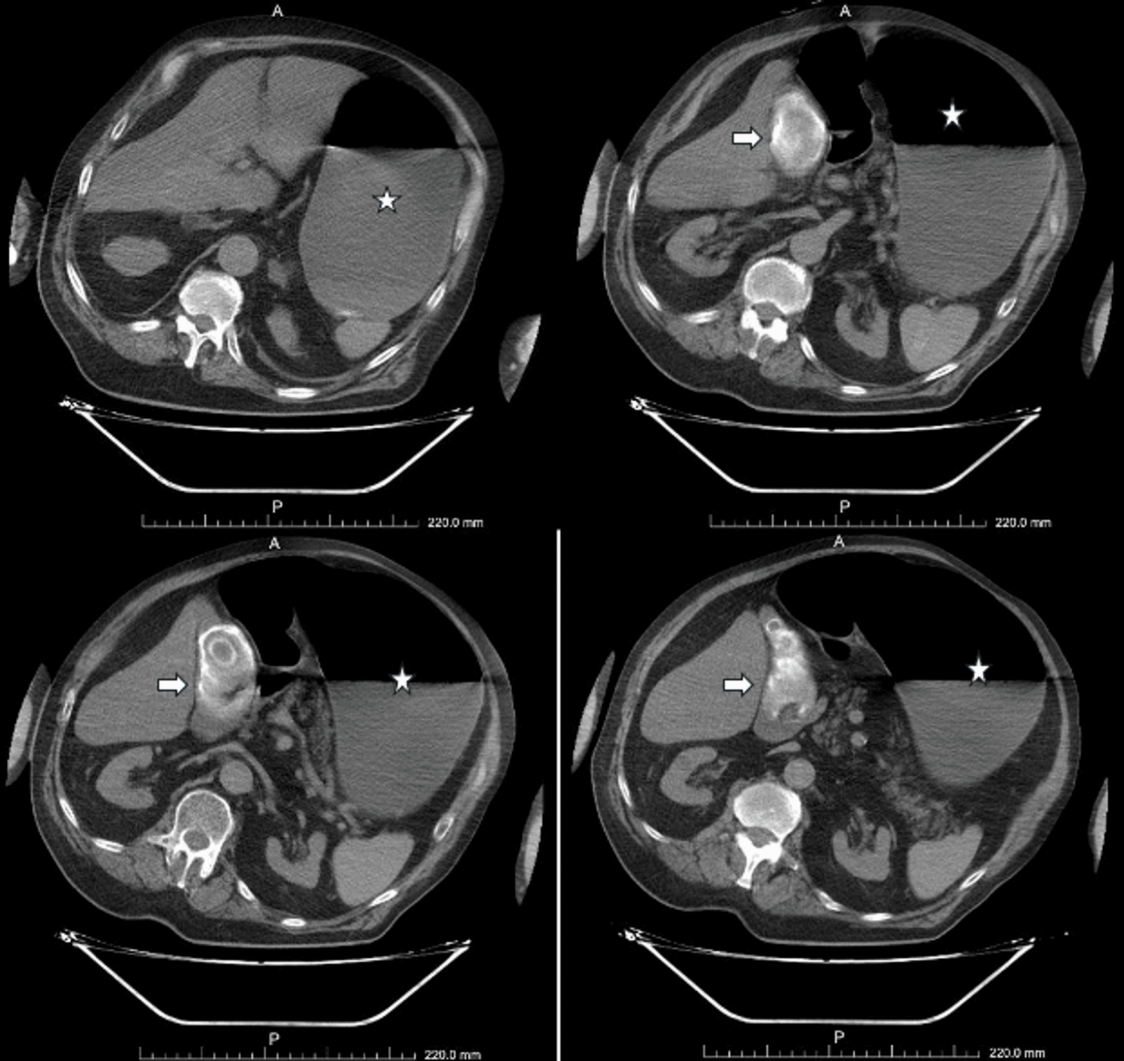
Preoperative abdominal computed tomography demonstrating marked gastric dilatation with significant gaseous and fluid distension. The arrow indicates extrinsic compression of the duodenum by the giant gallstone within the gallbladder. The asterisk (*) marks the markedly distended stomach.
